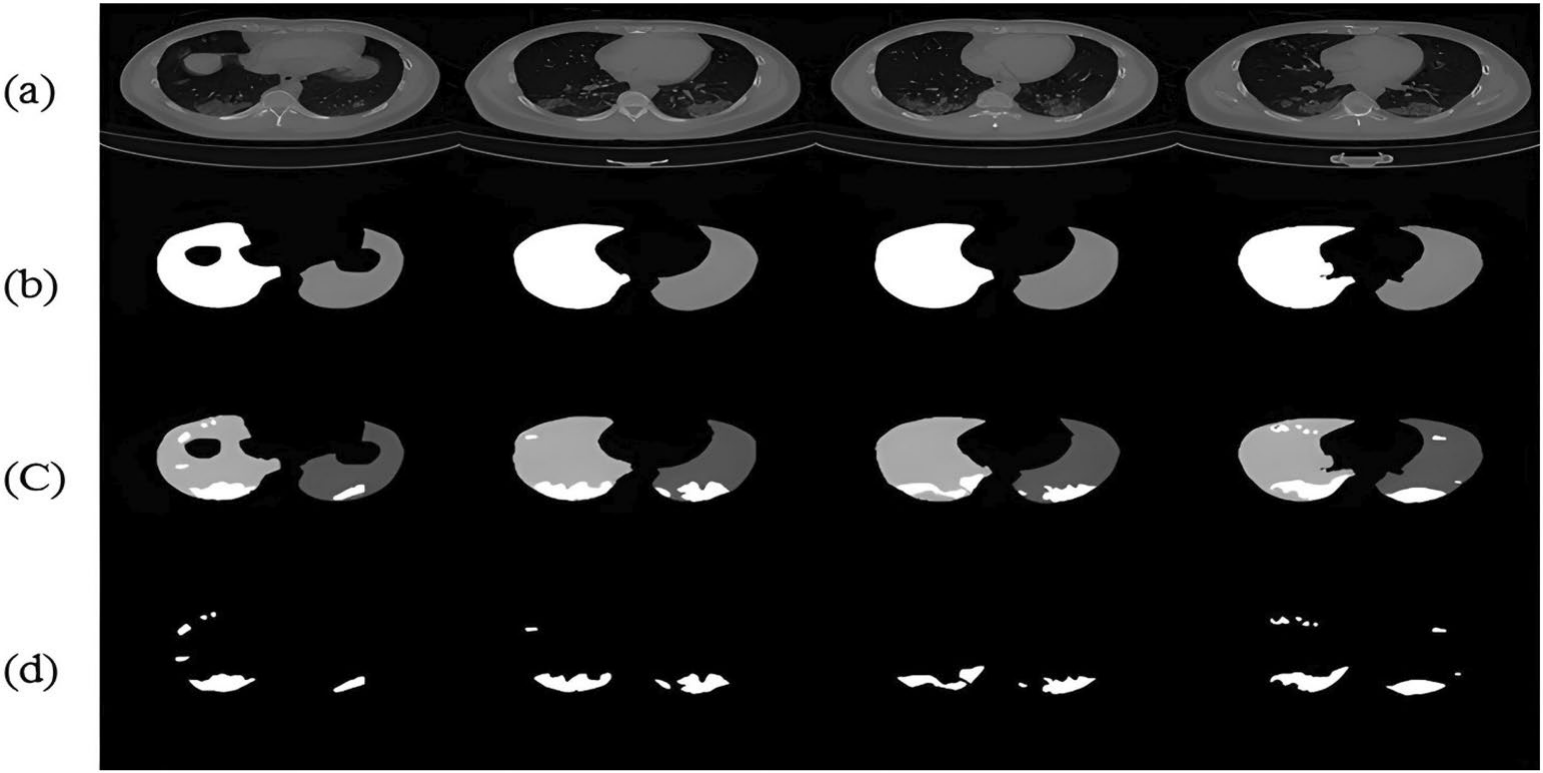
Four sample images from the used dataset. First row (a): original CT lung images. Second row (b): sample of lung masks. Third row (c): sample of infection and lung mask together. Fourth row (d): infection masks.
